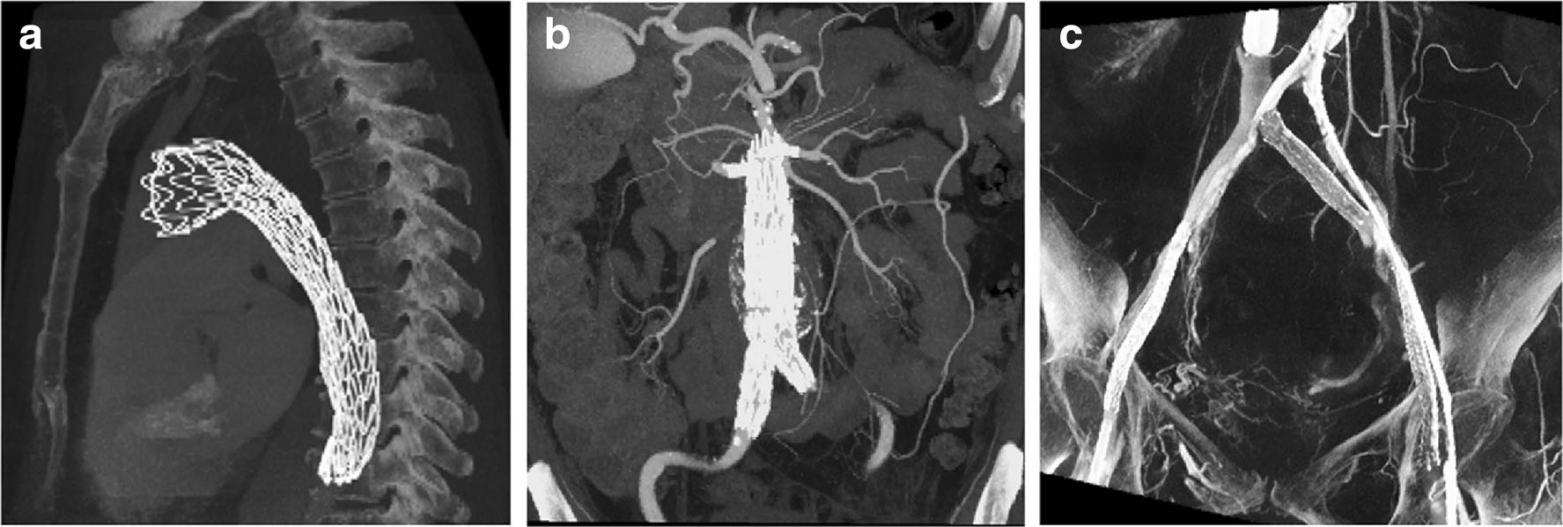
Human data sets: 3D high-resolution computed tomography data of a a thoracic aorta, b abdominal aorta, and c iliofemoral veins after endograft implantation
